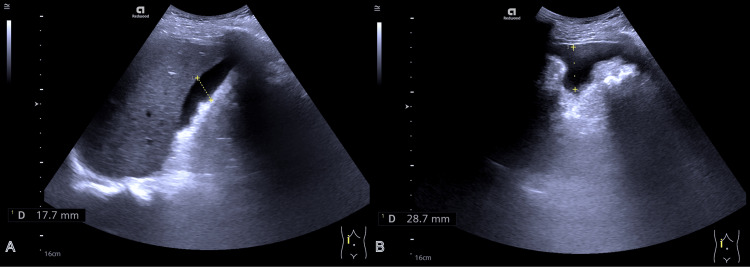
Ultrasound exploration of the abdomen detected a small amount of fluid around the liver (dotted line; A-B).
